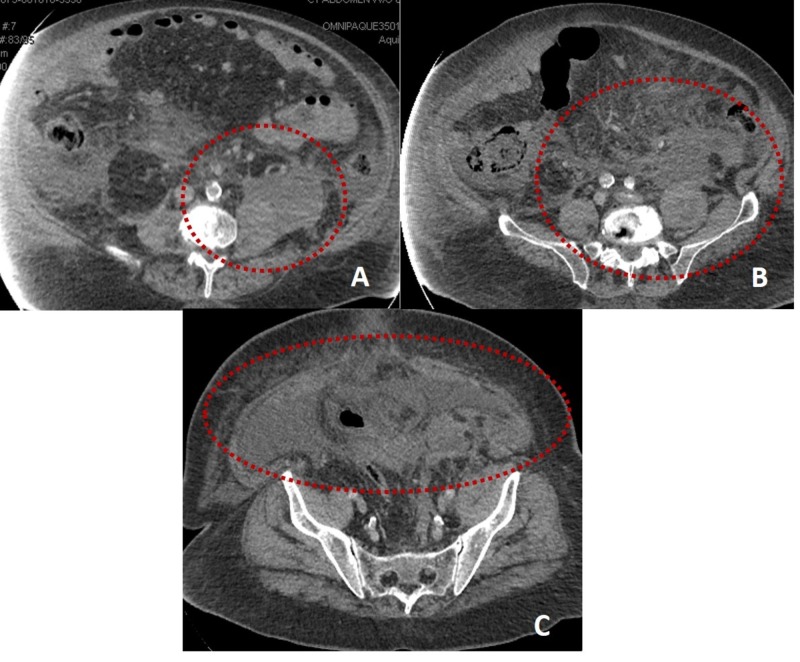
Computed tomography of the abdomen showing hyperdense fluid primarily within the left retroperitoneum, with extension into the lower right retroperitoneum. Hemorrhage centered in the left posterior pararenal compartment with possible extension into the substance of left psoas muscle. Panel A-C: Axial view showing different levels of the hemorrhage
